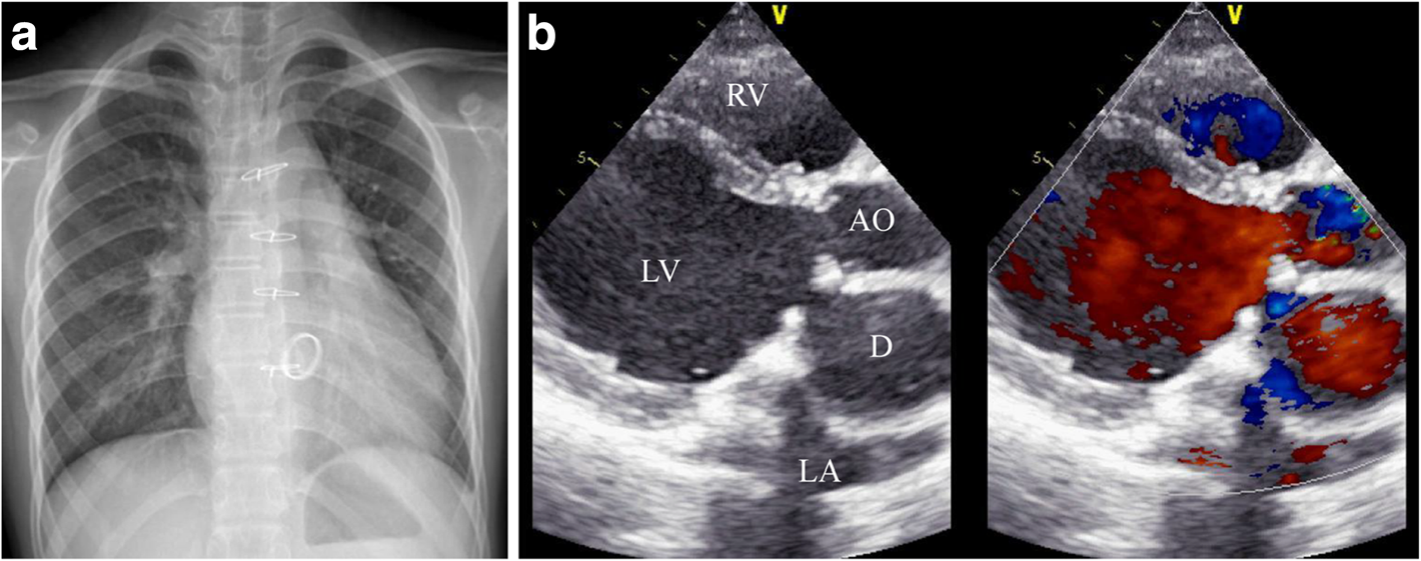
Multimodality imaging of the relapse left ventricular diverticulum (D). a. Chest x-ray film shows cardiomegaly. b. Transthoracic echocardiography shows a saccular structure (D) localized between the left atrium (LA) and the aortic root (AO), communicating with the left ventricle (LV) through a 13 mm wide neck
